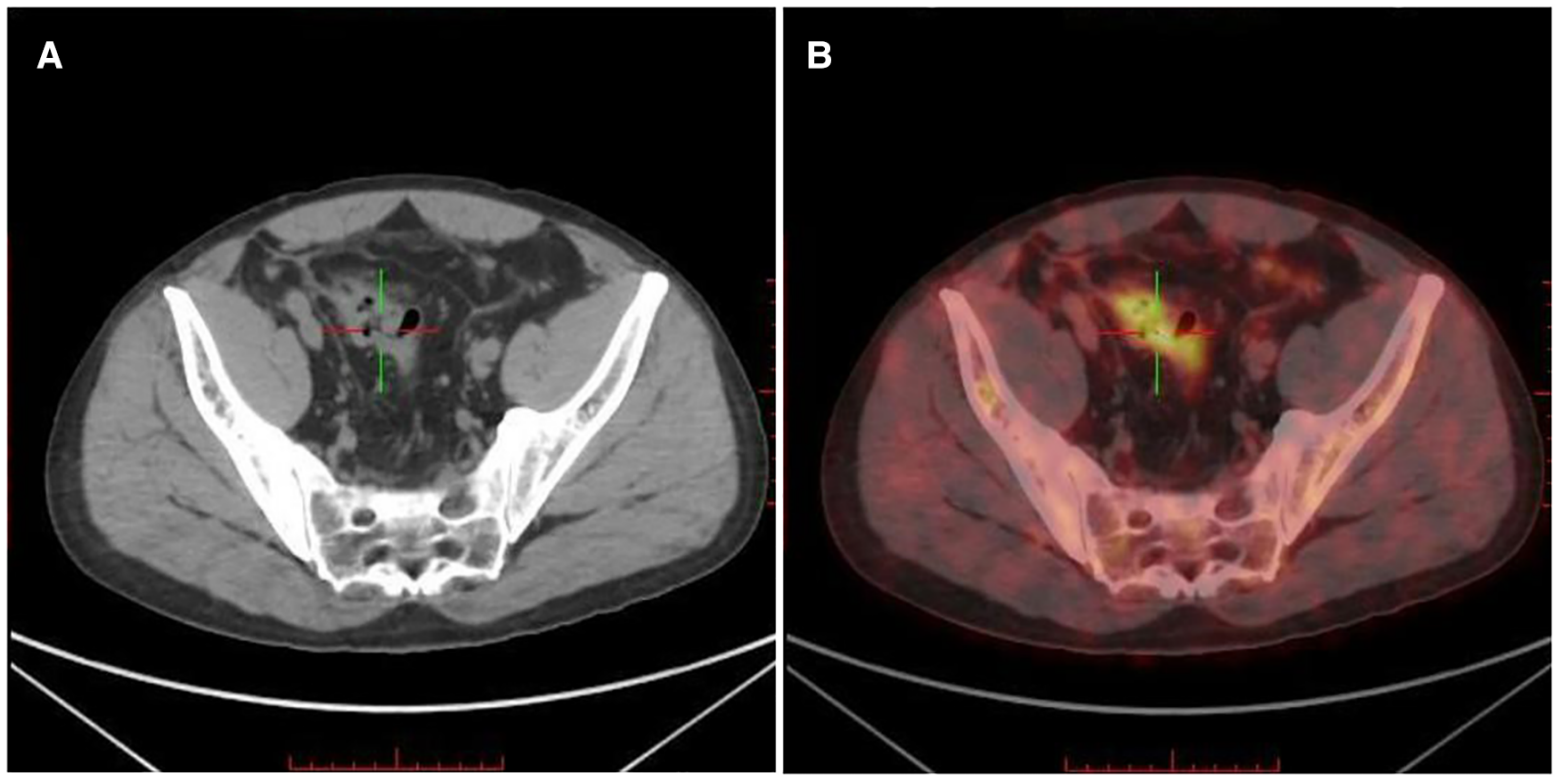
Positron emission computed tomography (PET-CT) of the lesion. (A) PET-CT showed a thickening of the sigmoid canal wall; (B) increased FDG uptake and a maximum SUV of approximately 7.8.
